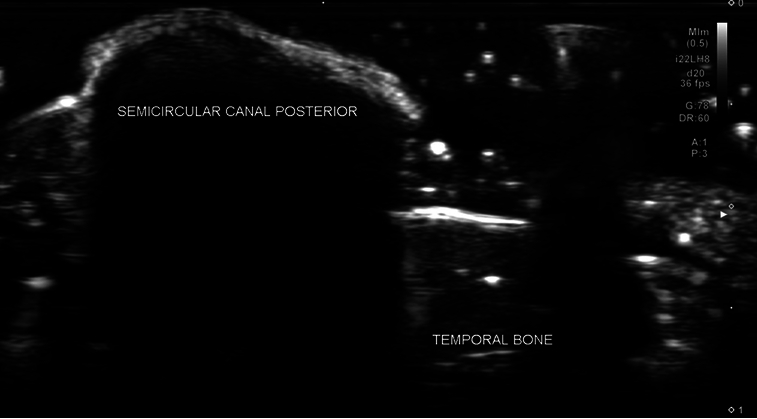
Sonogram (22 MHz) demonstrating differentiation between the echotexture of the semicircular canal and the temporal bone.
